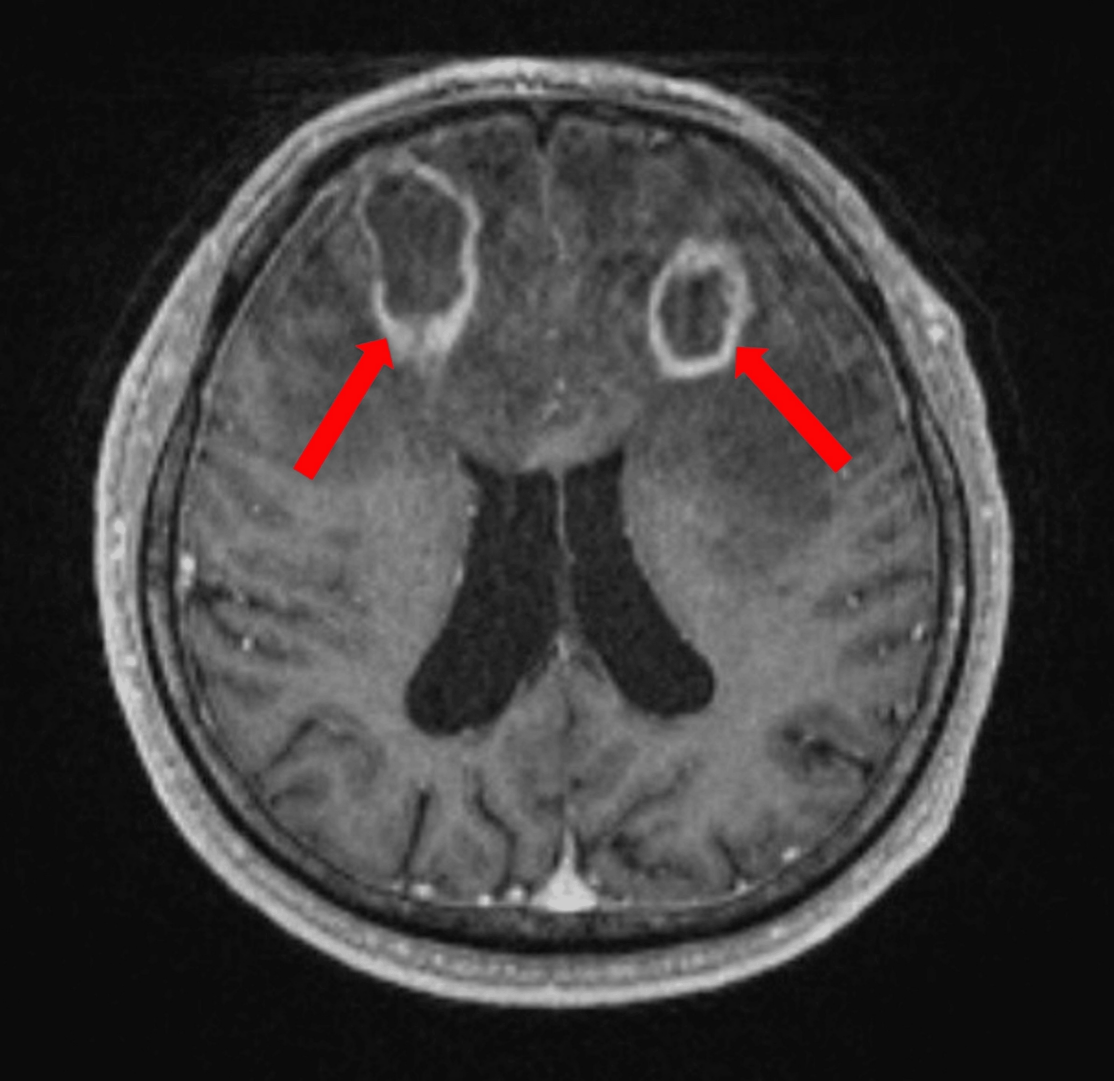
MRI of the brain without contrast Notable for two prominent peripheral rim-enhancing and diffusion-restricting lesions associated with extensive surrounding edema MRI: Magnetic resonance imaging
